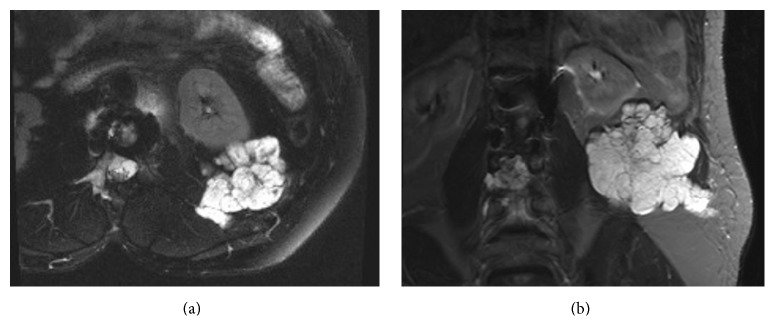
(a and b) Cross and Coronal sections MRI images show tumor recurrence with extension to psoas muscle.
